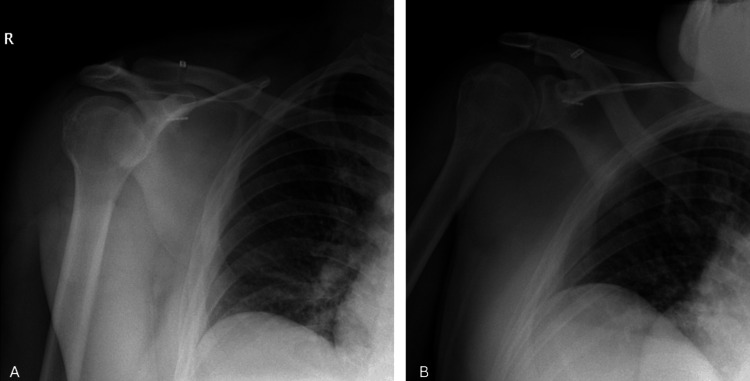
Postoperative radiographic images of the patient (A) AP shoulder radiograph. (B) True AP shoulder radiograph. Both images confirm correct implant placement and adequate fixation.
